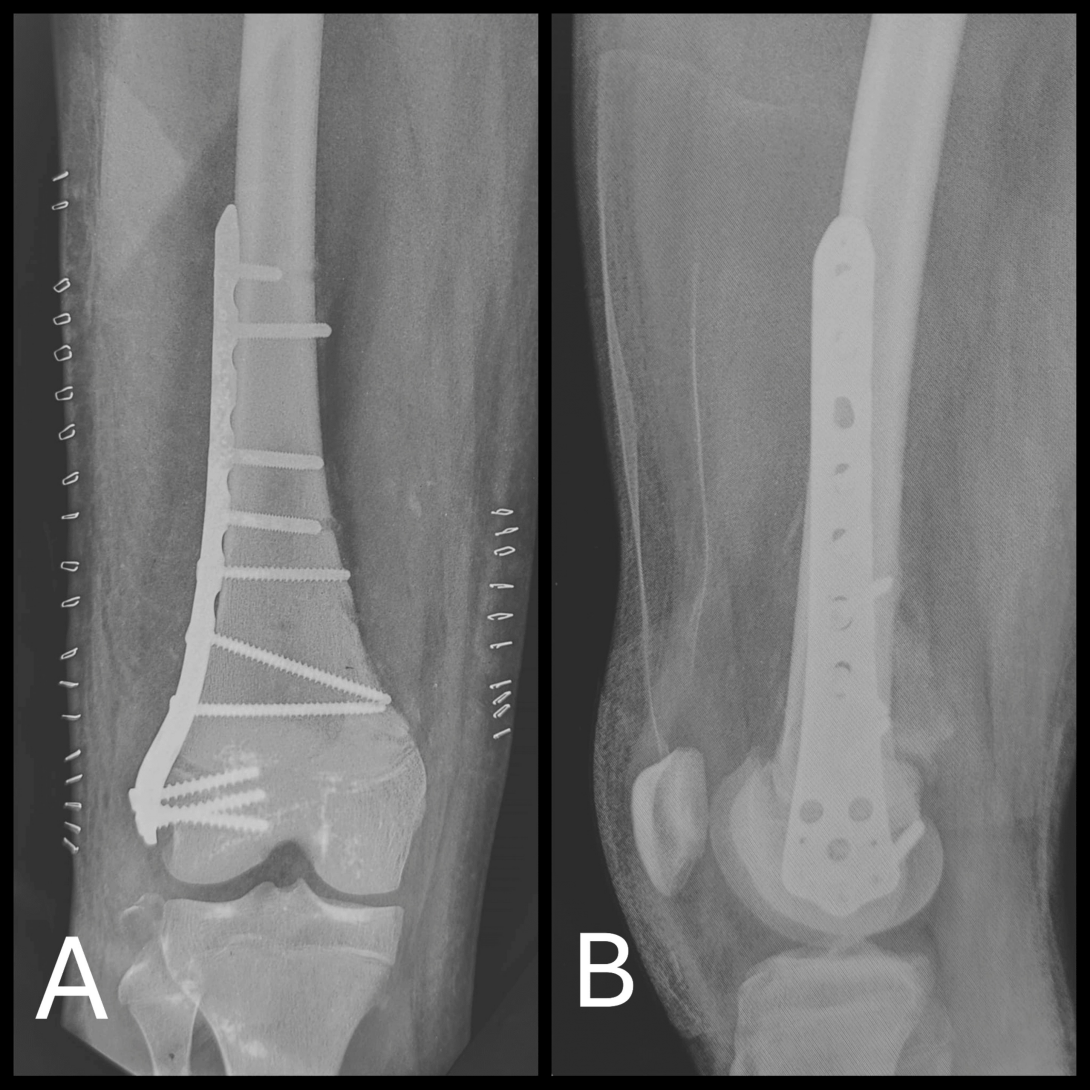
Post-op radiograph following osteochondroma excision and bony fixation. (A) Anteroposterior view; (B) lateral view
